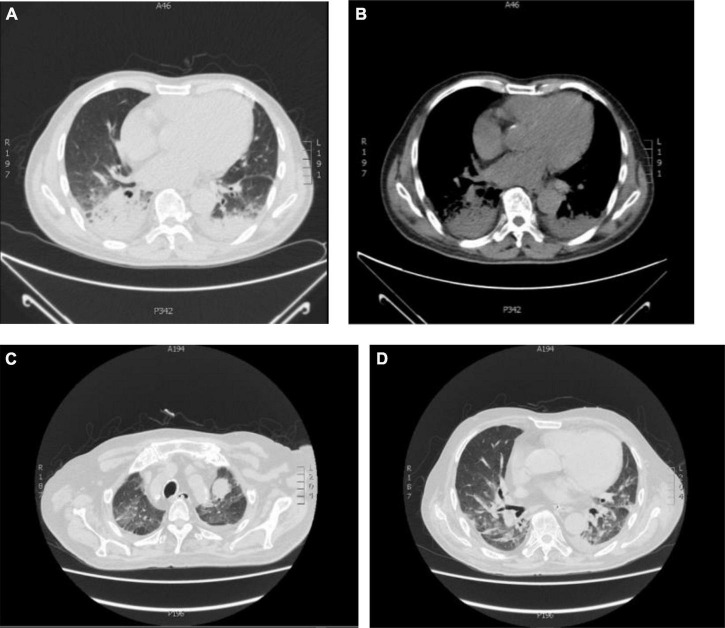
Chest CT imaging findings in two admissions. (A,B) Dense patchy shadows in the dorsal segment of bilateral lobes and a small amount of pleural effusion on the right; (C,D) chest CT of this admission (the diagnosis of Nocardia infection). Bilateral interstitial inflammatory changes. Old lung and pleura lesions. Limited emphysema in both lungs. Left pleural effusion. Heart enlargement, pericardial effusion, and left neck and left lung apex mass shadow.
